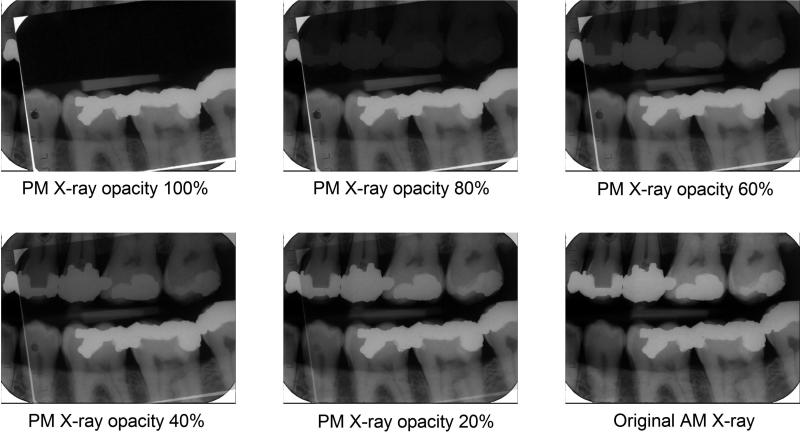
X-ray superimposition. Superimposing the images demonstrates the similarities between the dental features in an intuitive way. (Image courtesy Health Support Queensland Forensic and Scientific Services (HSQ FSS) and the Queensland State Coroner, with permission).
